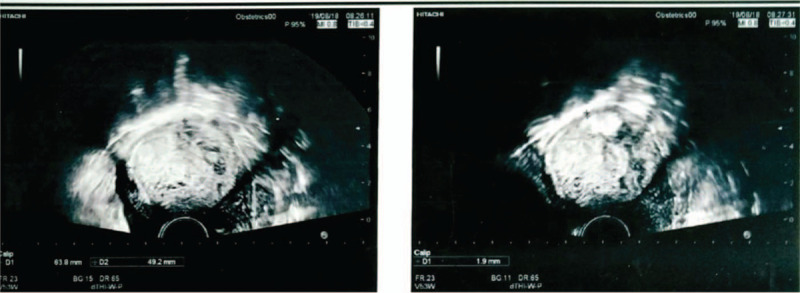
On the third day after curettage, ultrasound revealed an uneven echo group of 6.4 × 4.9 × 5.4 cm in the bottom of the right uterine cavity, which was not clearly demarcated from the posterior wall. The muscle layer became thinner, with a thinnest part of about 0.19 cm. Punctate blood flow signals were observed.
